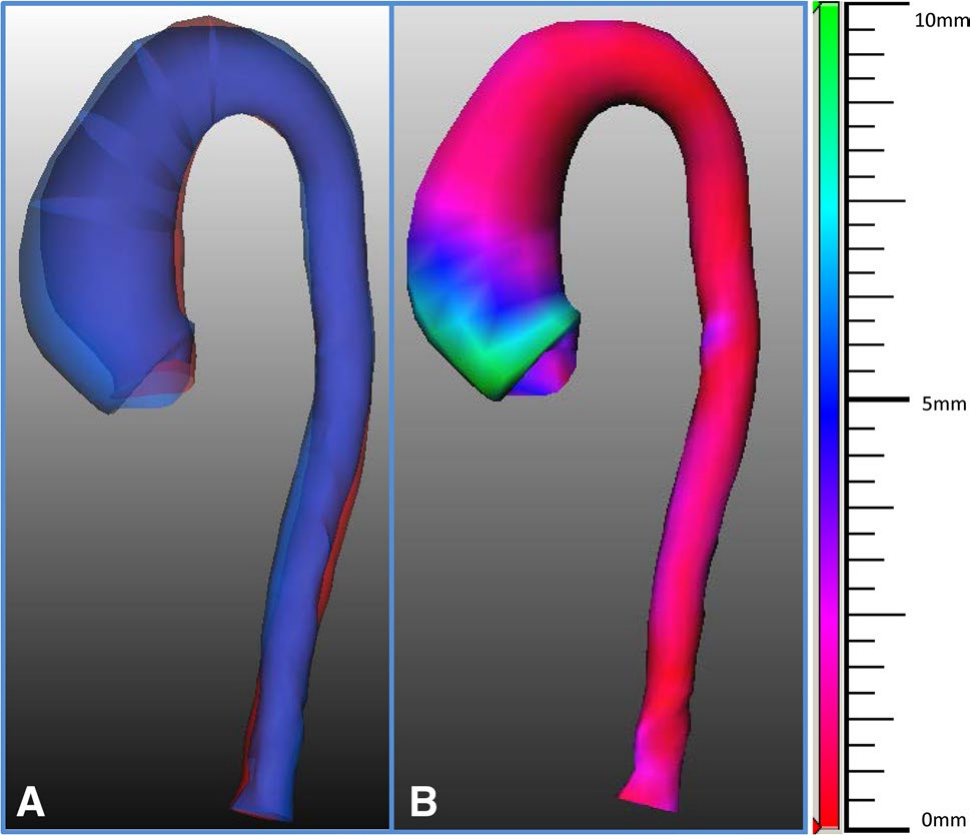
Tools for the visualization of the size changes in the aorta. In a superimposed three dimensional views of the surfaces of the thoracic aorta based on the semi-automatically segmented contour of both baseline (in red) and follow-up (in blue) CTA images. In b the semi-automatically calculated changes in diameters between the baseline and follow-up scans are represented with colors (red, blue and green indicate 0, 5 and 10 mm differences in diameters, respectively) for an immediate and comprehensive overview of the results. (Color figure online)
